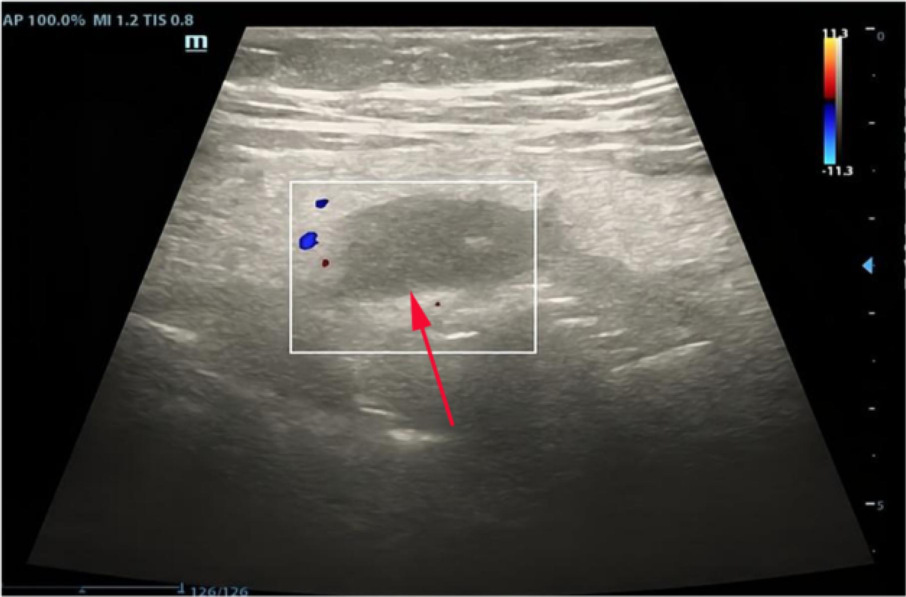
Initial abdominal ultrasound in the left lower quadrant. The greater omentum appears thickened with an internal hypoechoic avascular area (arrow), raising early suspicion of omental ischemia in an atypical left-sided acute abdomen.
